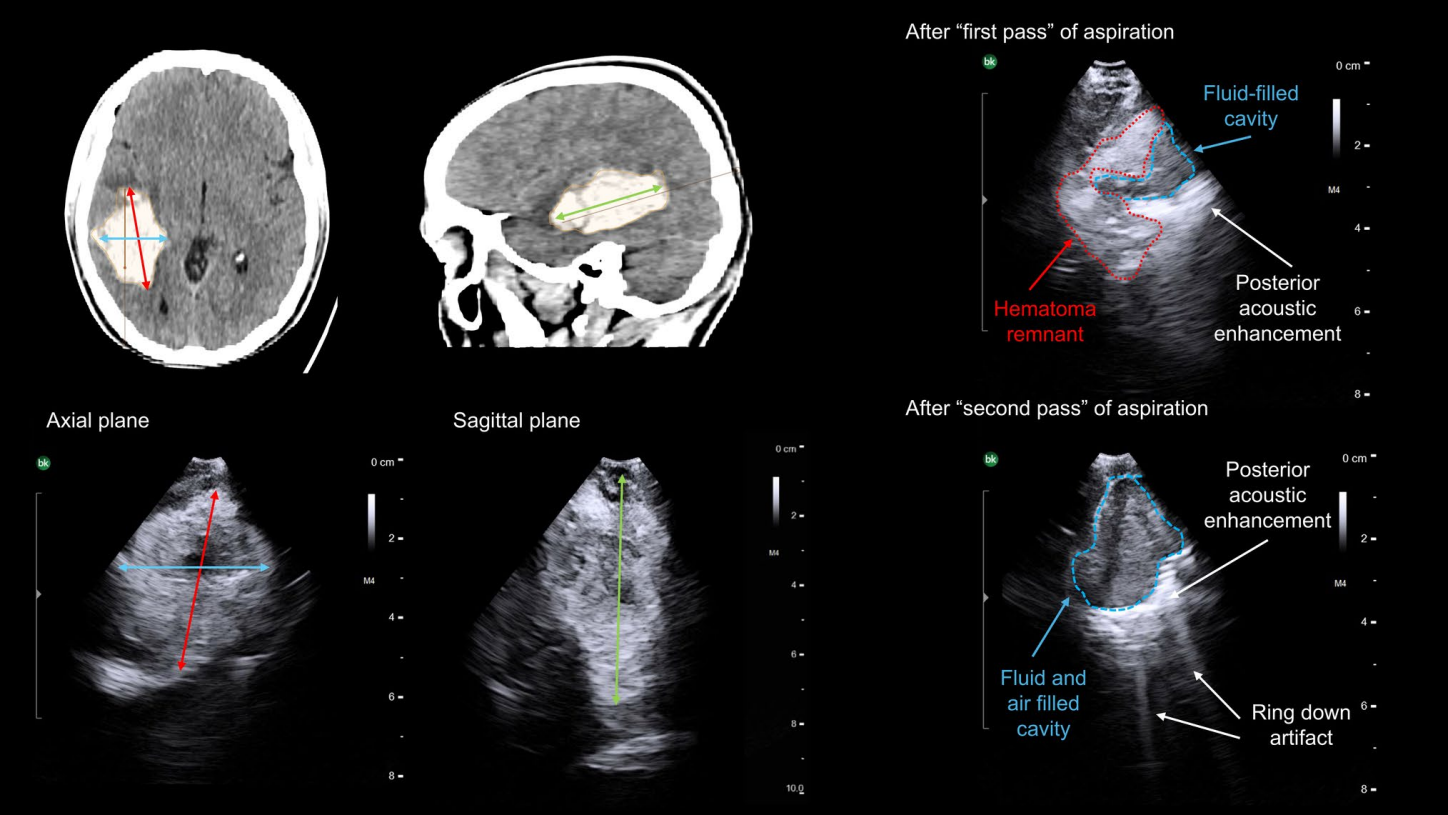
Hematoma remnant after a first pass of aspiration as detected with intraoperative US. The basal CT scan (axial and sagittal) of a patient with a 47 cm3 right temporal hematoma is shown on the upper left. The initial volume is calculated intraoperatively with US as shown in the lower left of the panel (red, blue and green representing the three dimensions of the hematoma). On the upper right, the US imagen after a first pass of aspiration is shown. Note that the cavity appears hypoechogenic, with a posterior hyperechogenic acoustic enhancement. The hematoma remnant can be delineated in the superolateral margin of the cavity, with an acoustic intensity like that at baseline. After a second pass of aspiration (lower right corner of the panel), the cavity appear hypoechoic, no clot remnants are seen. Note the posterior acoustic enhancement due to the presence of liquid (saline) in the cavity. Also note the ring-down artifact (“irregular linear hyperechogenic lines”) provoked by the presence of air within the cavity
